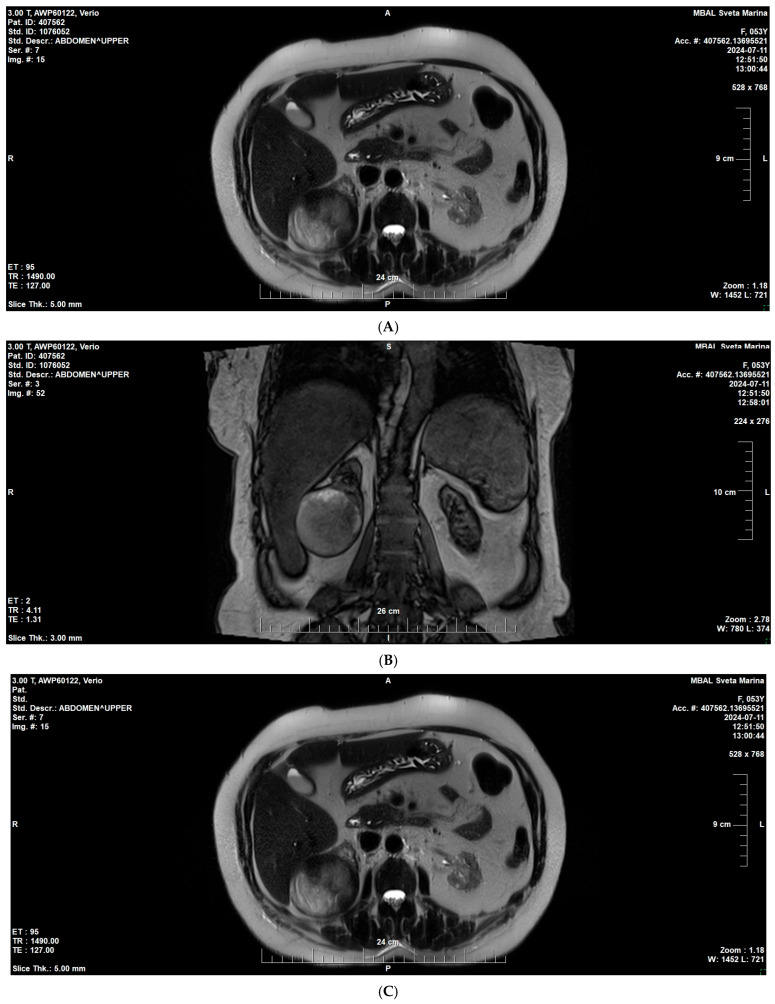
MRI of the native right kidney (July 2024). (A) Coronal T1-weighted image showing a well-circumscribed exophytic mass in the lower pole of the right native kidney (59 × 67 × 68 mm). (B,C) Axial T2-weighted images demonstrating heterogeneous signal intensity.
